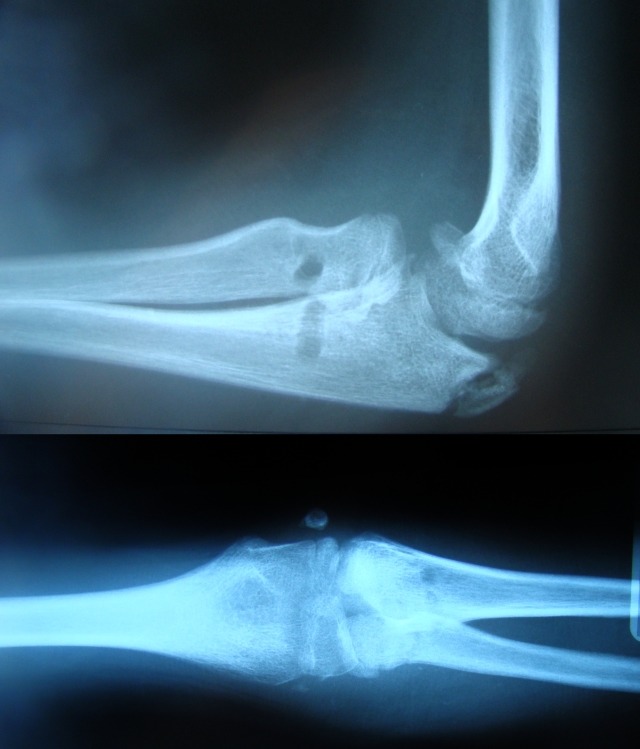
Radiologic aspect 30 days after surgery. There are two radiolucent areas corresponding to the tunnel through the radial neck and the point where the ulnar interference screw is inserted. The lateral X-ray, with the elbow flexed, shows a slight dislocation of the radial head in relation to the ulna; this position is required by the technique in order to avoid the constriction of the radial neck and subsequent progressive limitation of pronation and supination. The anterior-posterior X-ray, with the elbow extended, shows a normal placement of the radial head in relation with the ulna and the humeral condyle.
